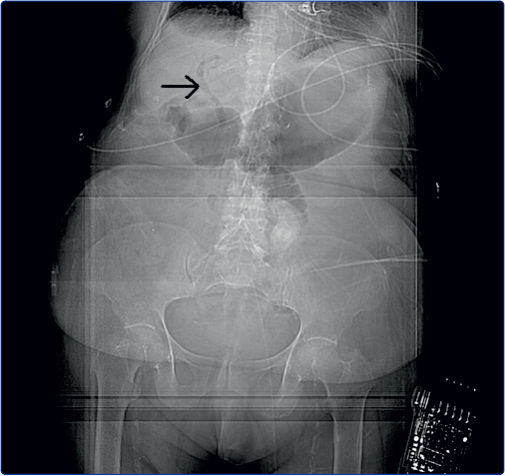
CT scan of the patient's abdomen showing pneumobilia and a choledochoduodenal fistula.
